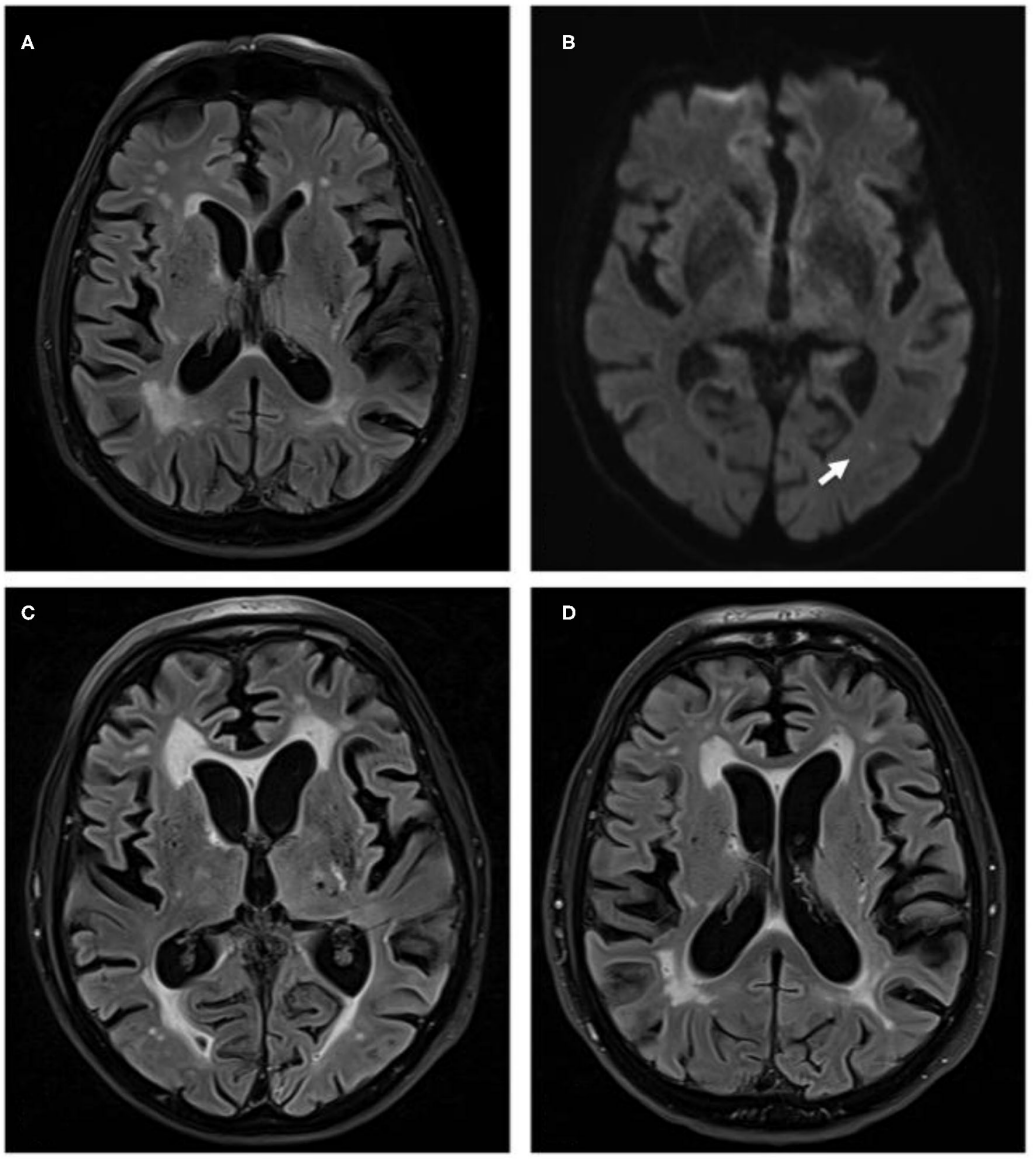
(A) Magnetic resonance brain images with fluid attenuated inversion recovery (FLAIR) sequences of October 2017, when patient presented with recurrent seizures. Images show distinct cerebral small vessel disease. (B) Diffusion weighted imaging sequences of the same examination show point-shaped infarction in left-sided MCA-territory (white arrow). (C) FLAIR sequence of April 2018 when patient deteriorated clinically with clear increase of white matter damage. (D) FLAIR sequence of October 2018, when patient presented for fifth cycle of cyclophosphamide treatment and with a marked clinical improvement. Images show a clear reduction of small vessel disease.
